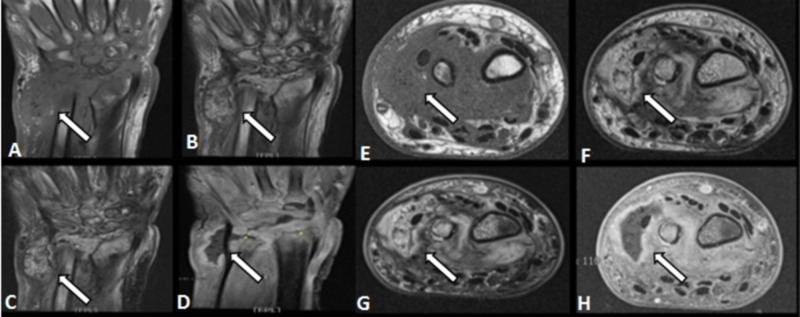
MRI film of the left wrist at the two months follow-up, suggestive of erosive arthritis with a gas-forming abscess along the ulnar side A-D : coronal view; E-H : axial view MRI : magnetic resonance imaging
